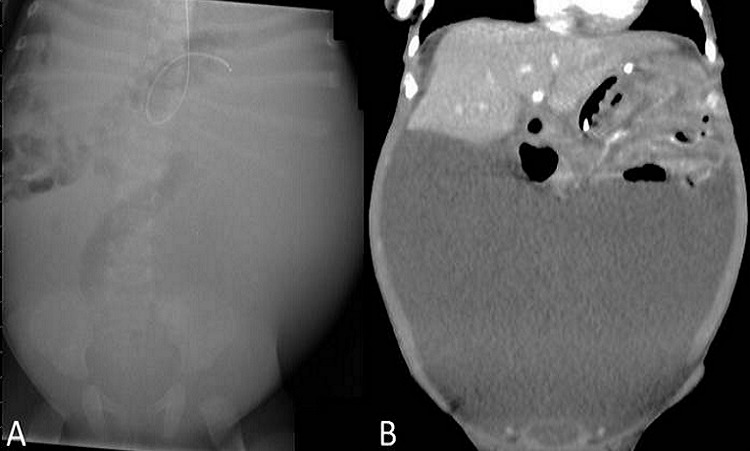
Figure 1: A: Abdominal x-ray showed a large opacity in the left side of the abdomen with associated displacement of bowel around it. B: In coronal CT scan a homogenous cystic mass was found filling most of the abdomen and displacing solid viscera and bowel.
